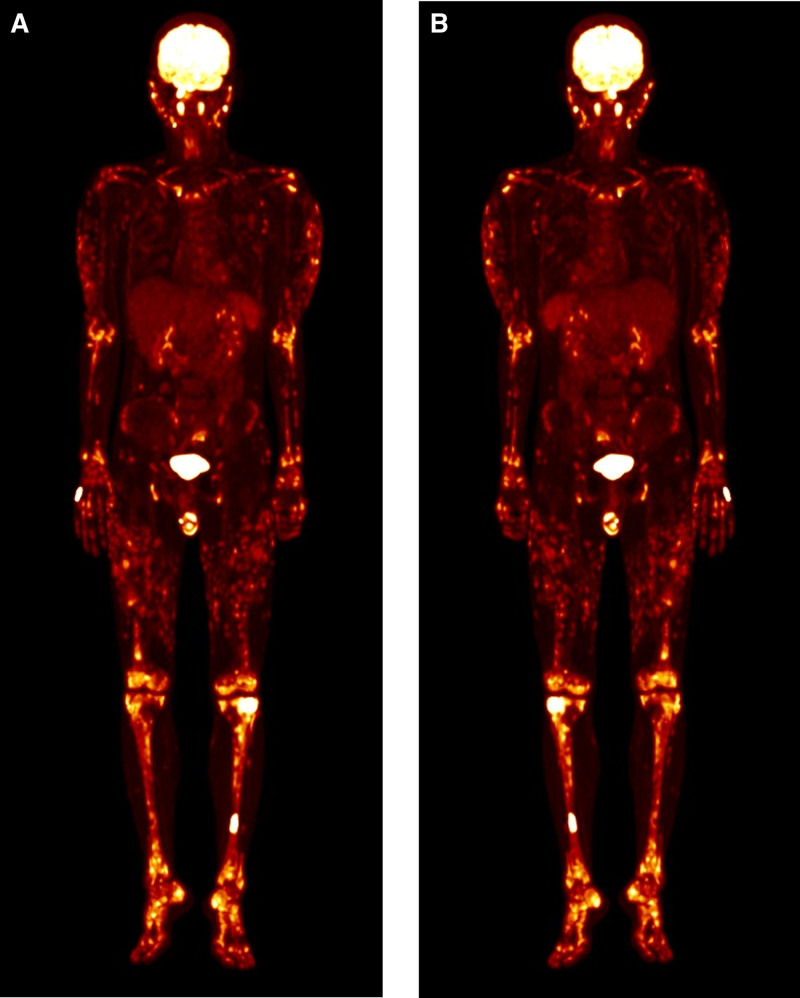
FDG-PET scan. (A) Front. (B) Back. FDG-PET scan showed probable lymphoma involvement including bilateral nasal cavity and left ethmoid sinus, multiple lymphatic chains both sides of the diaphragm, subcutaneous lesions, bones, and spleen. FDG-PET = fluorodeoxyglucose-positron emission tomography.
